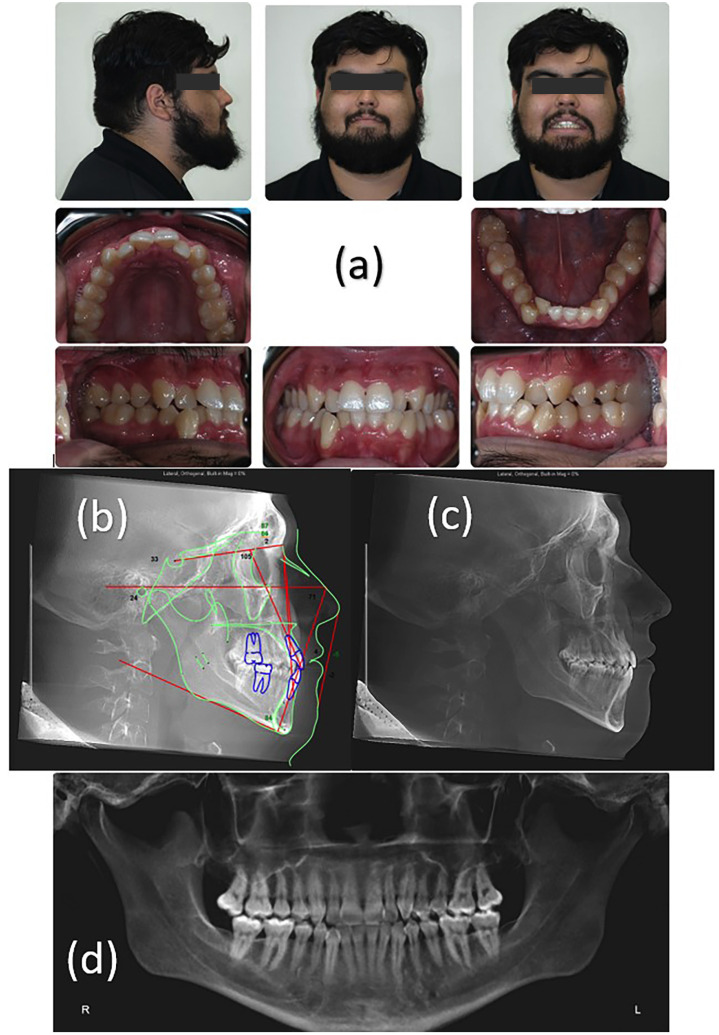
(a) Pretreatment facial and intraoral photographs, (b) Pretreatment lateral cephalogram, (c) tracing, (d) panoramic radiograph.
